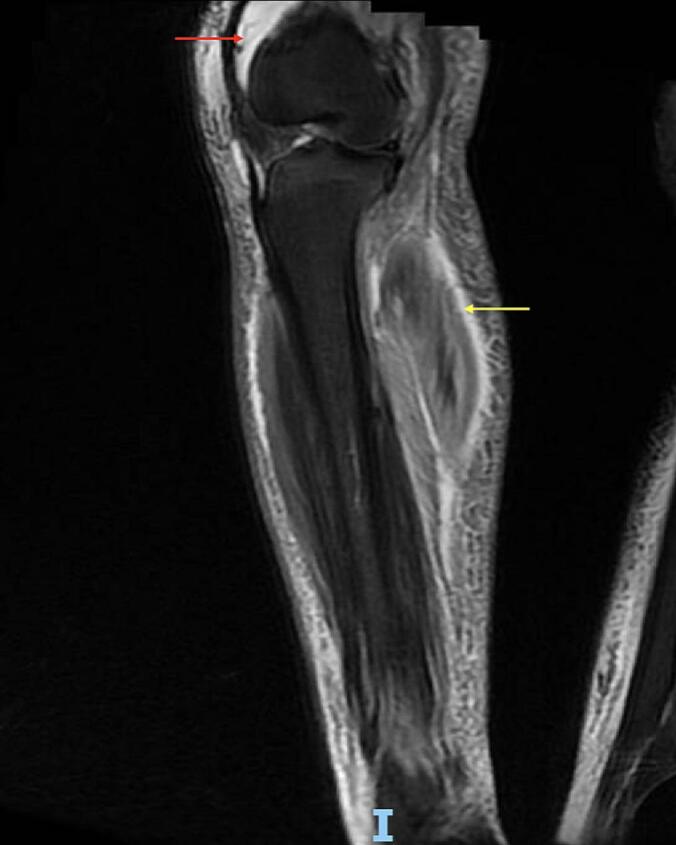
Sagittal T2-weighted MRI of Patient 1's right knee. The red arrow indicates a moderate suprapatellar joint effusion. The yellow arrow highlights a hyperintense signal within the posterior compartment musculature, overlaid by subcutaneous oedema and dermal thickening, consistent with soft tissue infection involving the fascia and muscle. (For interpretation of the references to colour in this figure legend, the reader is referred to the web version of this article.)
